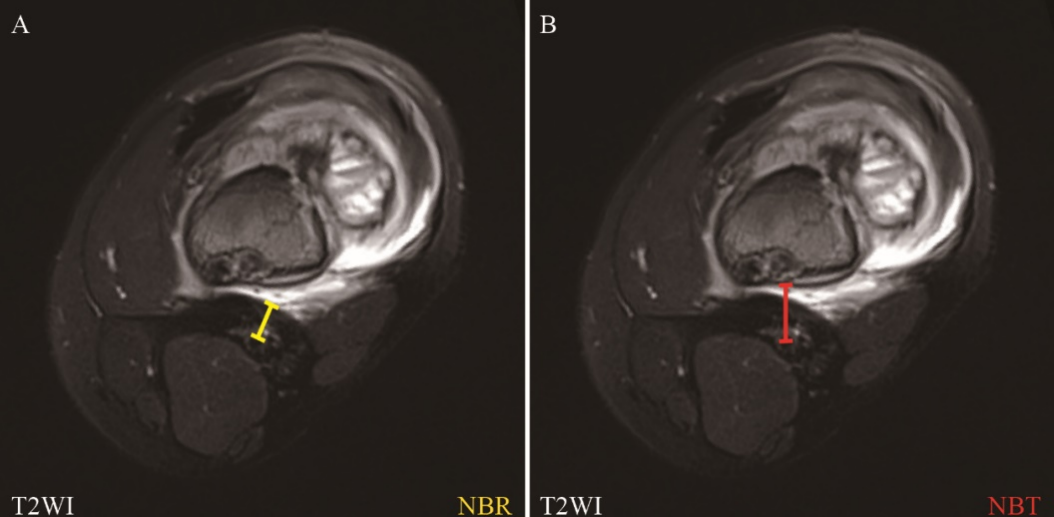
Assessment of the nearest of blood vessels to reactive zone (NBR) and the nearest of blood vessels to the tumor (NBT) in MRI. NBR: the nearest of blood vessels to reactive zone; NBT: the nearest of blood vessels to the tumor.
